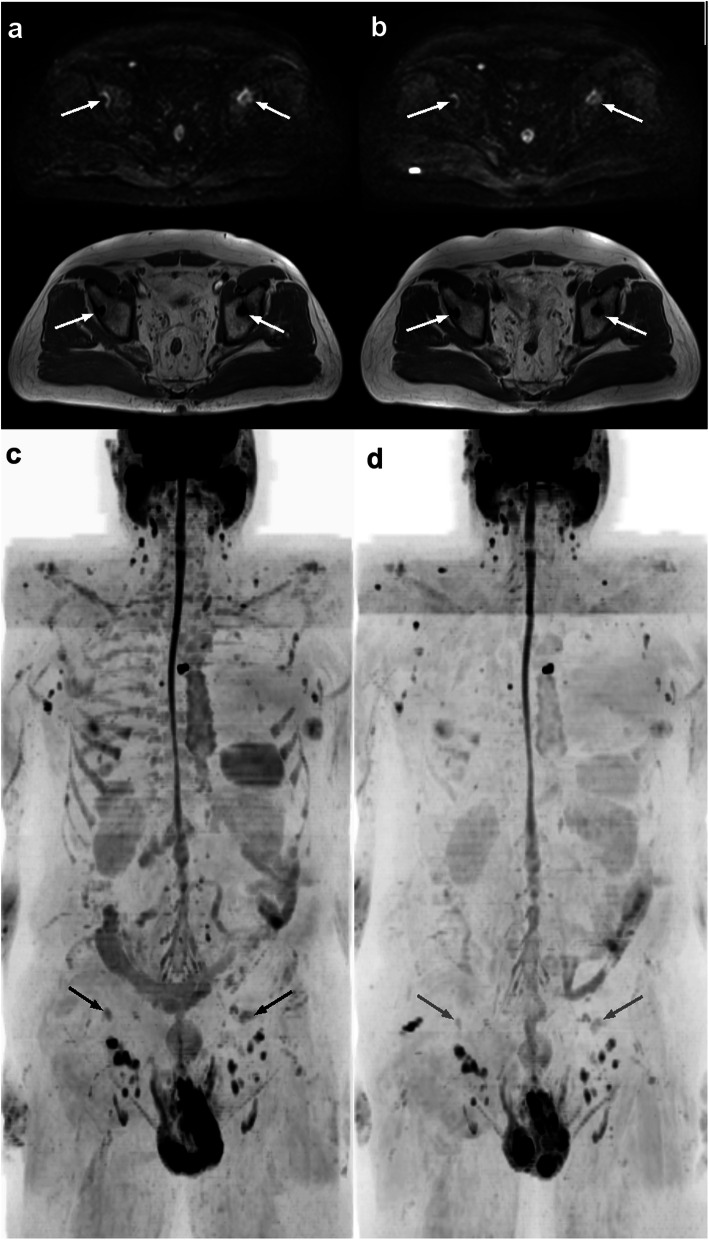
Example images of a 76-year-old man with advanced prostate cancer that is not progressing, with primary/dominant RAC 3. a) Axial DW b900 images (upper) and T1 images (lower) show the presence of lesions in pelvic bone (arrows) that are unchanged in b) follow-up MRI. The c) initial and d) follow-up b900 maximum intensity projection images confirm stability of disease throughout the body
